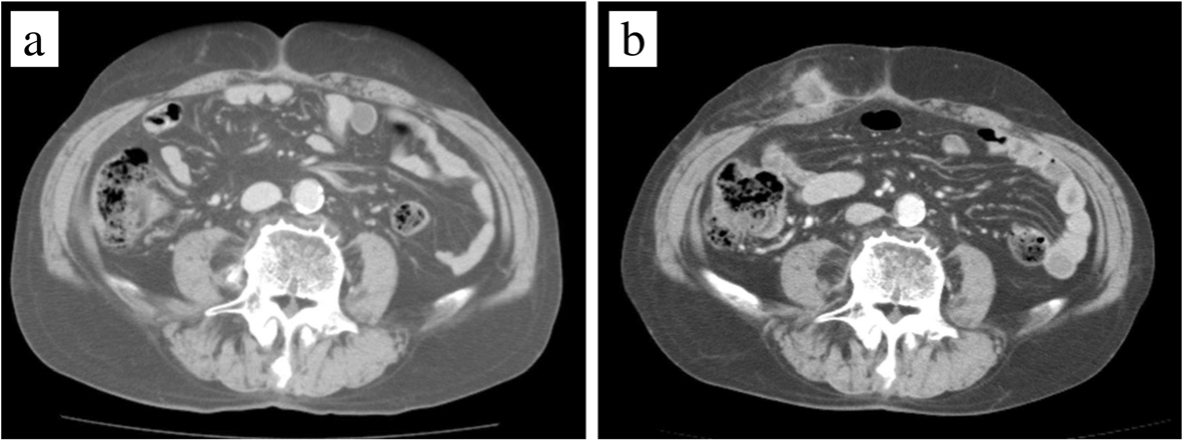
An abdominal contrast-enhanced computed tomography (CT). a Two months before the initial diagnosis of Schloffer tumor. b Pre-operative CT scan showed a ring-enhanced mass at the ventral side of the right abdominal wall
